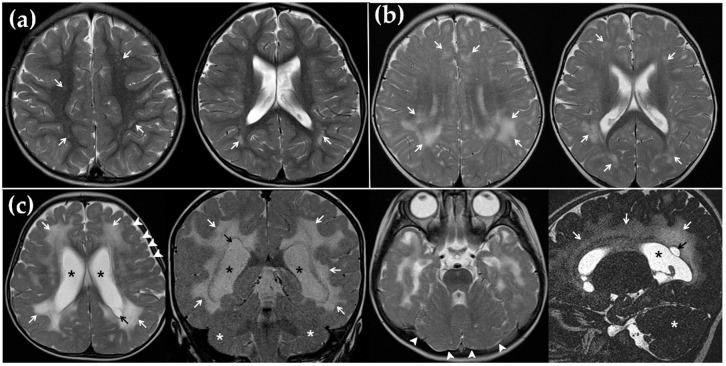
Various features of T2-weighted MR images in patients with cytomegalovirus infection. (a) Multifocal white matter (WM) lesions (white arrows) in deep WM of frontal and parietal lobe (CH-4). (b) Extensive WM lesions (white arrows) in periventricular and deep WM of whole brain areas (CH-9). (c) Diffuse WM lesion (white arrows) combined with periventricular cyst (black arrow), ventriculomegaly (black asterisk), cerebellar hypoplasia (white asterisk), and polymicrogyria (white arrowhead) (CH-3).
